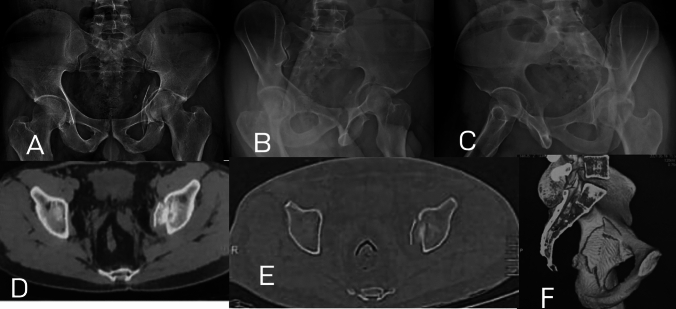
Pure quadrilateral plate fracture; A AP view, B Iliac Oblique view showing intact posterior column, C Obturator Oblique view showing intact anterior column with elevated plate of the quadrilateral surface, D, E;Axial CT cuts and F; 3D reconstruction showing displacement of the quadrilateral surface with intact both columns
